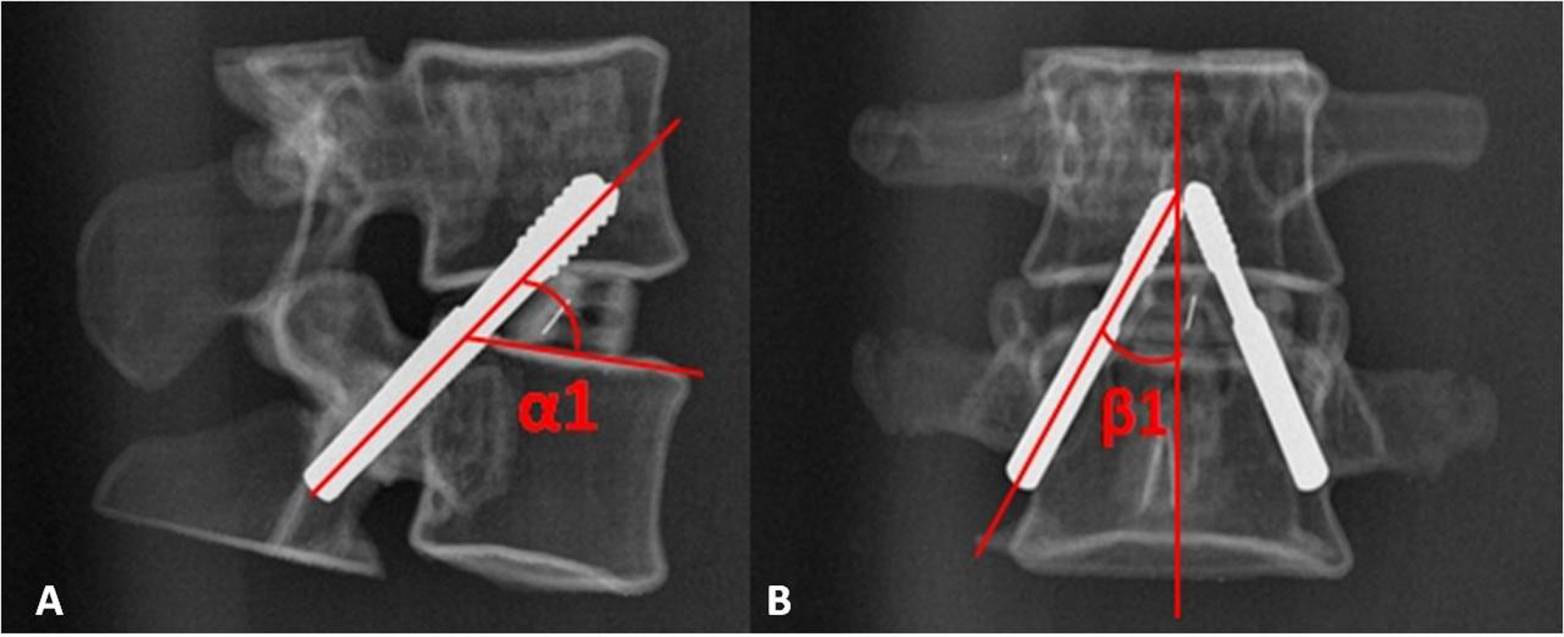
The radiographic lateral and anteroposterior (AP) films of the 3D printed lumbar model were obtained after the TPTD screw was introduced. “α1” is the angle between the TPTD screw and the upper endplate of the lower vertebra measured on radiographic anteroposterior films on the AP films; “β1” is the angle between the TPTD screw and the vertical centerline was measured radiographic lateral films.
